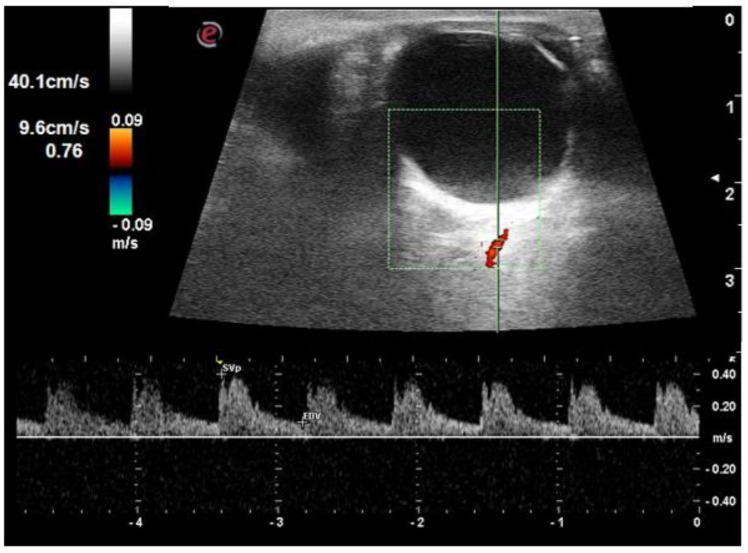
An orbital Doppler image in a patient with diabetes without retinopathy shows a resistive index of 0.76.
